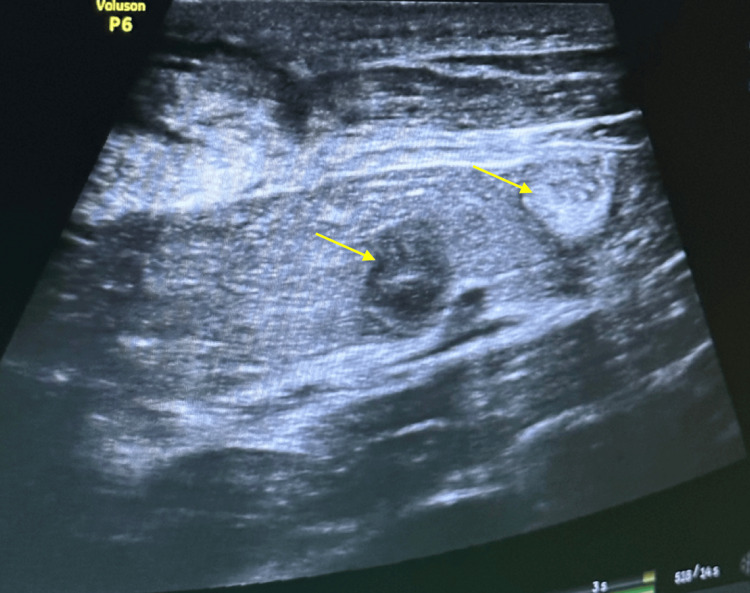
Ultrasound image showing two hypoechoic nodules indicated by yellow arrows in segment II of the liver (arrows).
